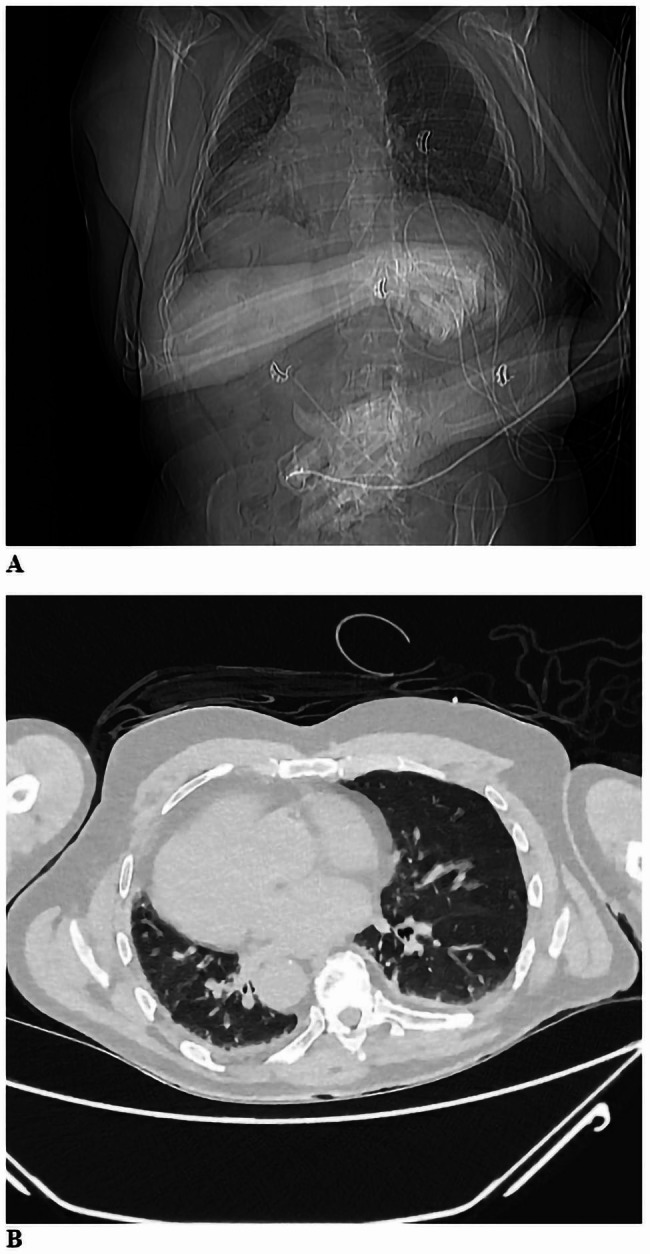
Situs inversus totalis. Situs inversus totalis on X-ray (A) and CT (B)
